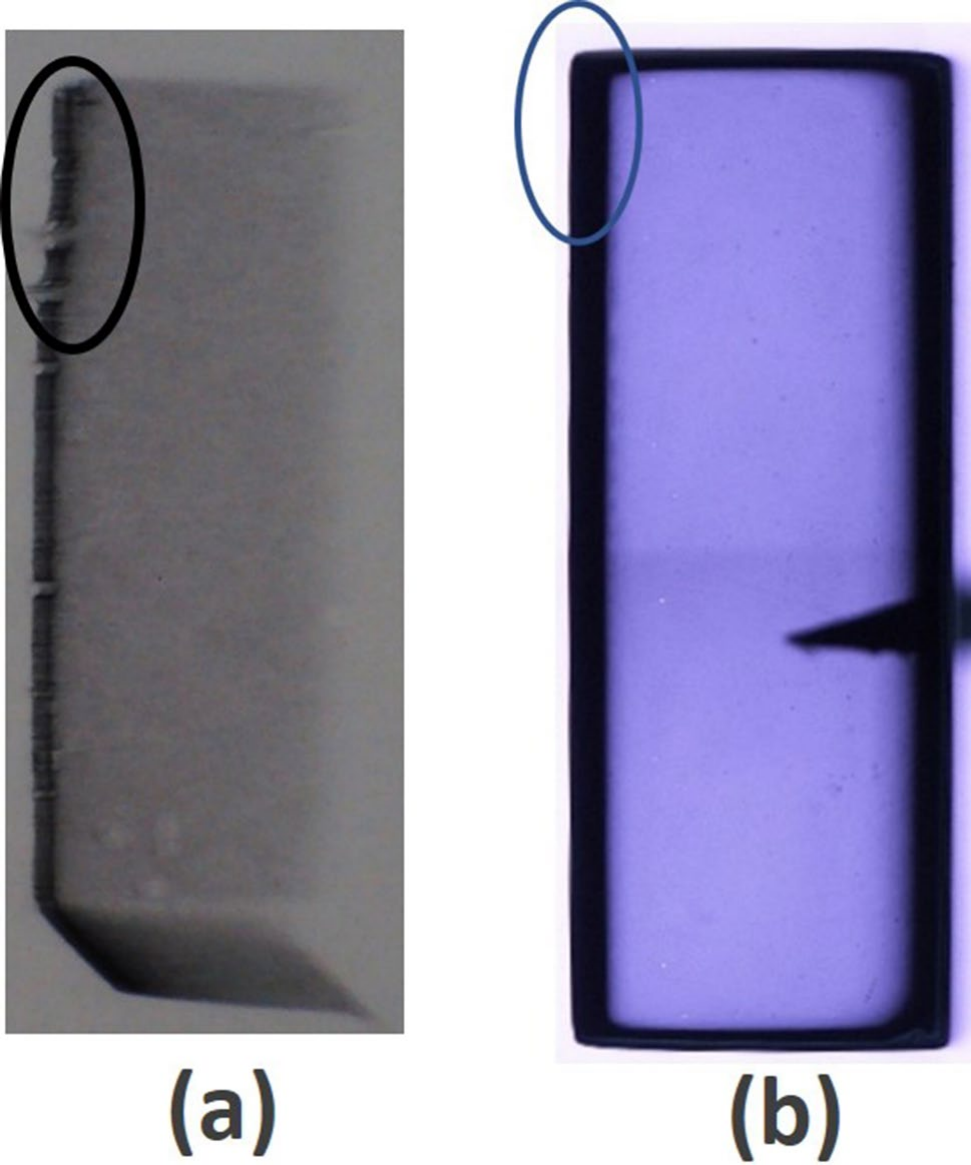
(a) X-ray topographic image and (b) IR transmission image of the as-grown Cd_0.9_Zn_0.1_Te_0.98_Se_0.02_ detector sample. Slight bending on the top left side of the sample is highlighted by the ellipse.
